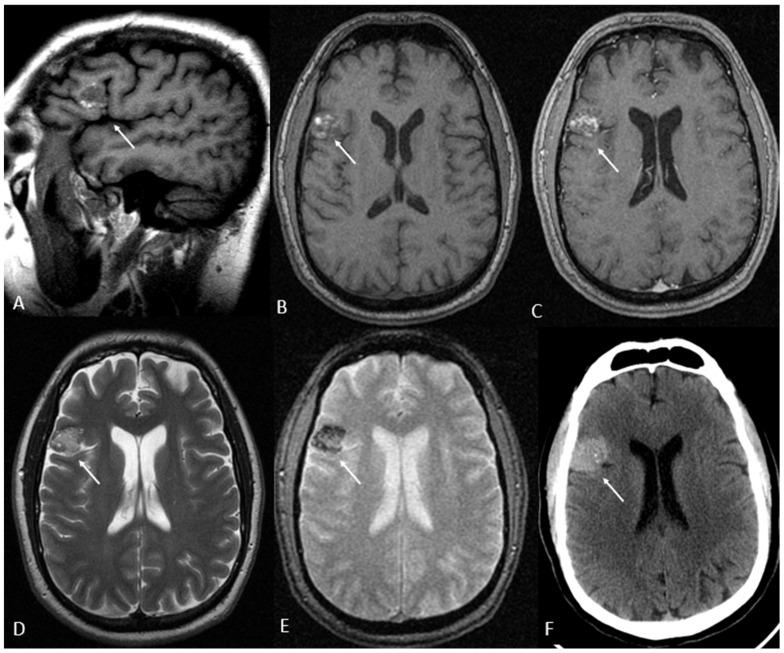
Right frontal opercular cavernoma on magnetic resonance imaging (MRI) (A–E) and computed tomography (CT) (F). T1-weighted sequences without intravenous contrast in sagittal (A) and axial planes (B), axial post-contrast T1-weighted (C), T2-weighted (D) and T2 GRE (E) images. There is an ovoid-like lesion in the right frontal opercular gyri containing hyperintense T1 and T2 areas, peripheral hypointense T2 rim, blooming artifact in T2 GRE and slight enhancement after intravenous contrast administration. In axial CT (F), the lesion corresponded to a hyperdense image with small punctiform calcifications. These findings are consistent with an opercular cavernoma (arrows). Note that no signs of acute complications were observed.
